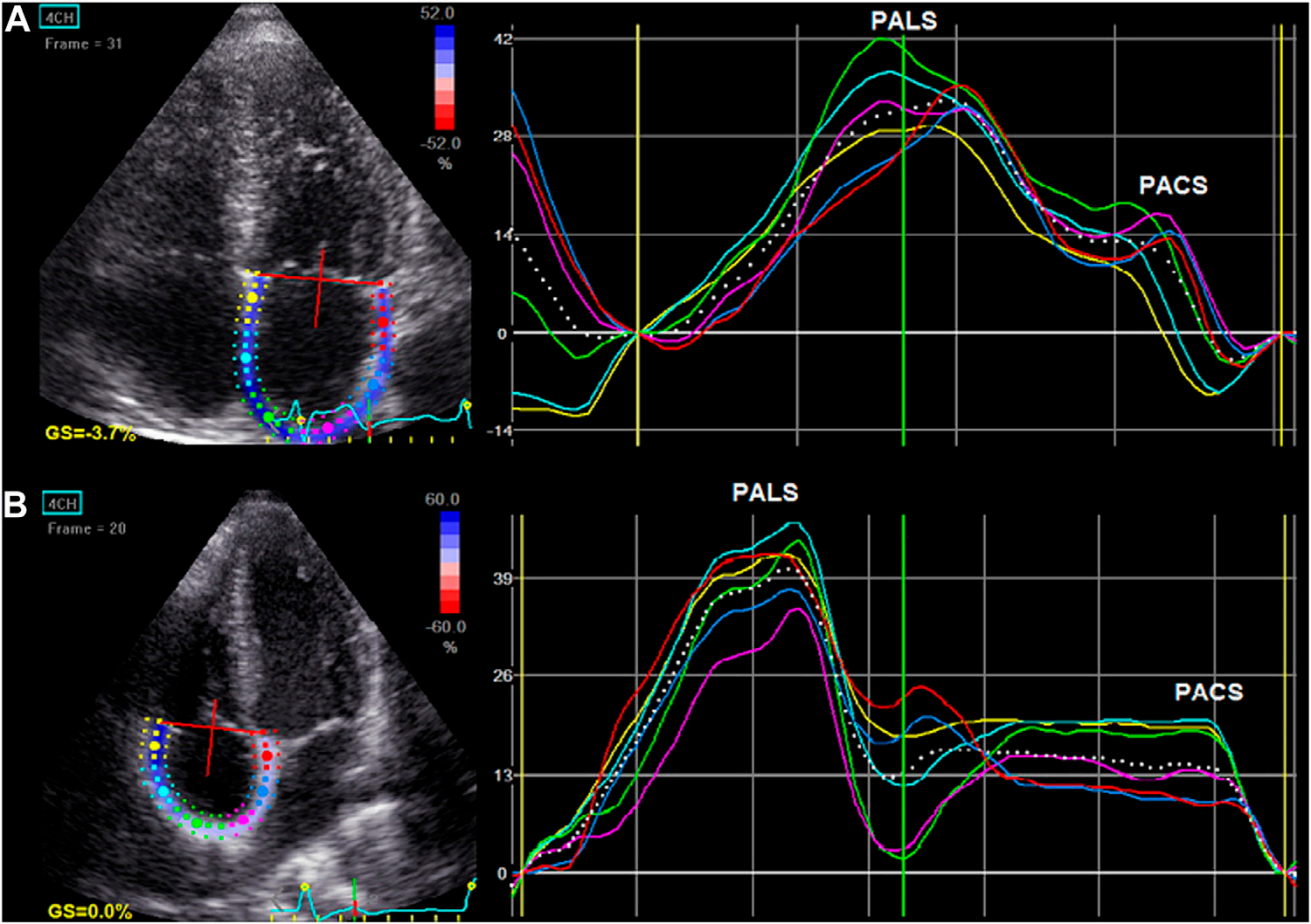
Left atrial (A) and right atrial (B) longitudinal myocardial deformation by two-dimensional speckle tracking echocardiography (2D STE). The dashed curve depicts the average strain. The first positive peak of the curve is the peak atrial longitudinal strain (PALS), measured at the end of the reservoir phase. The second peak, just before the active atrial contraction, represents peak atrial contraction strain (PACS).
